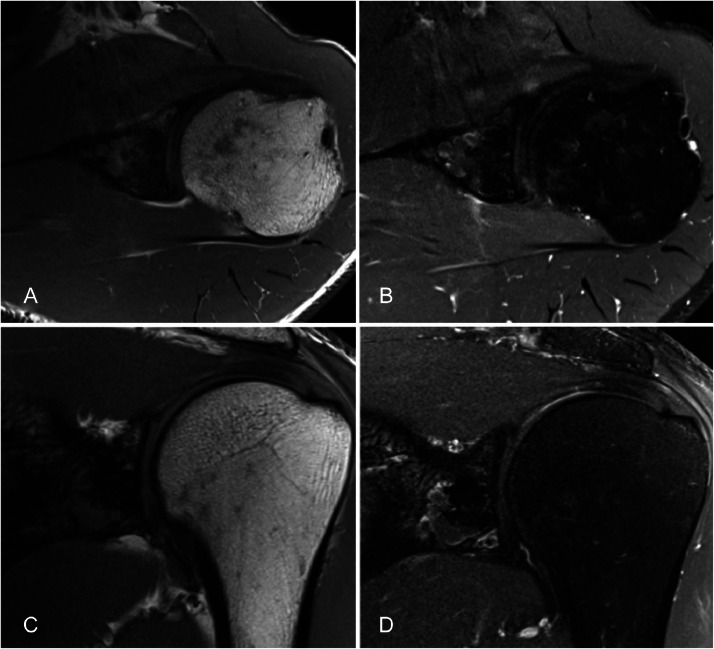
MRI of the left shoulder with (A) T1-weighted axial, (B) T1-weighted fat-saturated post gadolinium, (C) T1-weighted coronal, and (D) T1-weighted fat-saturated post gadolinium coronal sequences. No enhancing component is demonstrated within the lesion.
